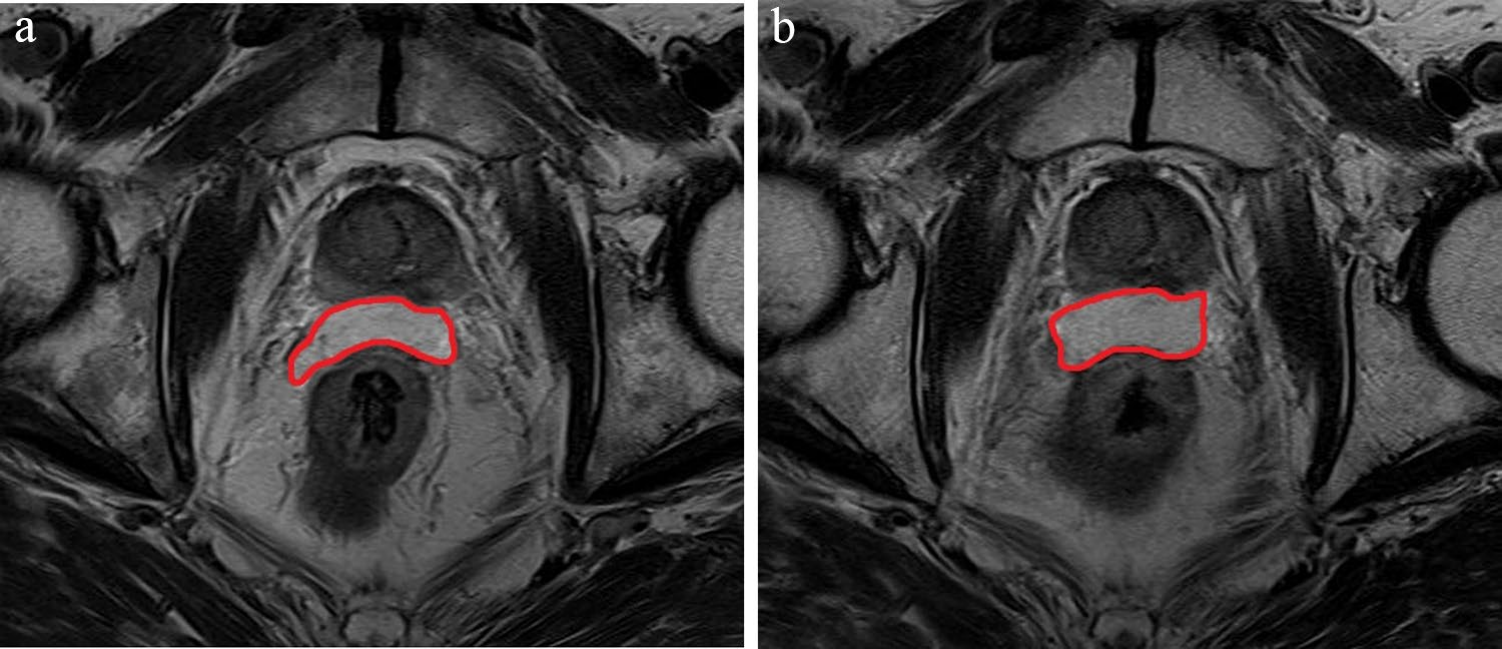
The hydrogel spacer contoured T2-weighted magnetic resonance images before (a) and during EBRT (b) from the same patient. The spacer volume obviously increased from 12.74 cc to 17.10 cc
